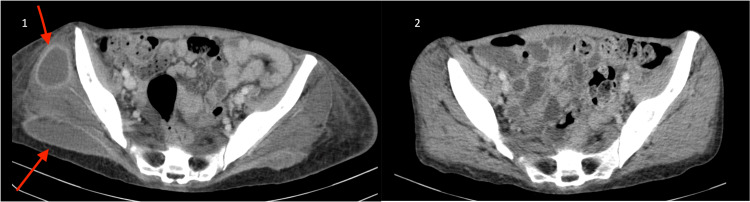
A CT scan was performed to evaluate the abscess in the gluteal region before and after drainage (1) A CT scan was performed before the drainage on the 40th day from the collection in the right gluteal region. (2) A CT scan was performed 60 days after discharge, showing no abscess in the gluteal muscle. The arrows show abscesses.
